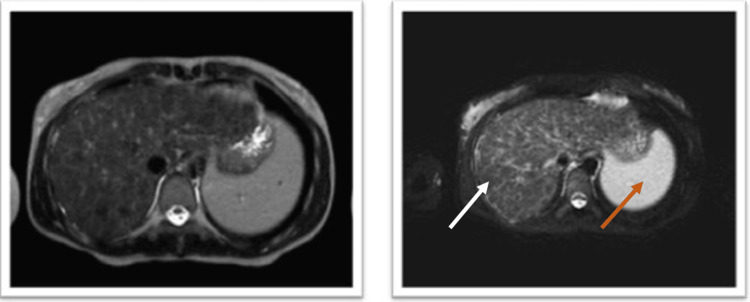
Patient's abdominal MRI revealing a liver with diffuse heterogenic nodular structure (white arrow) and mild splenomegaly (orange arrow).
